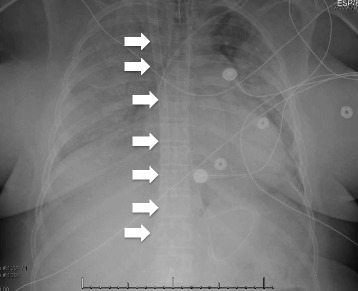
Veno-venous cannulation for ARDS. This chest X-ray demonstrates severe airspace disease in a patient with ARDS. The dual-lumen ECMO cannula (*arrows*) can be seen passing through the internal jugular vein, superior vena cava, and terminating in the inferior vena cava at the level of the hepatic vein
